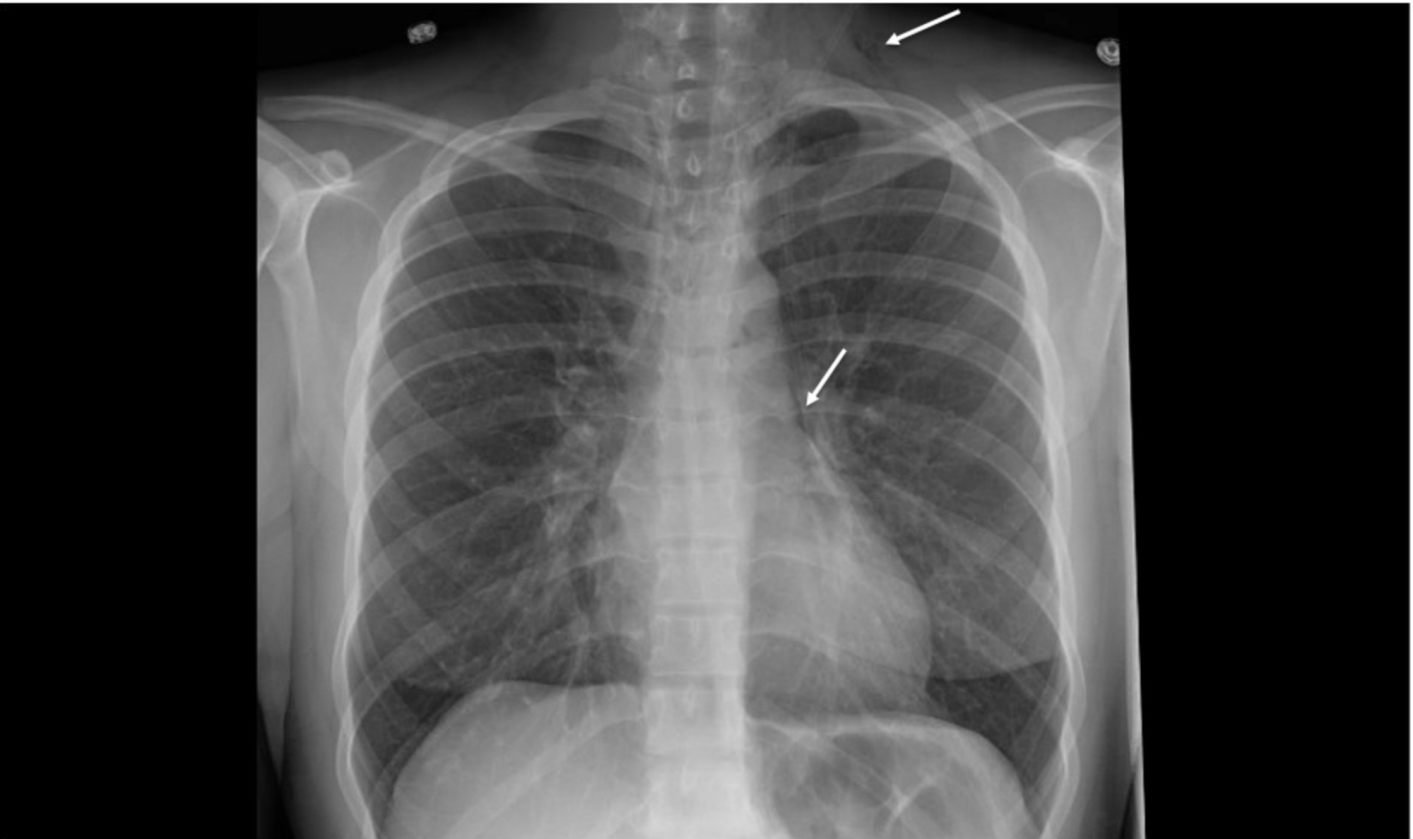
Plain chest radiograph demonstrating pneumomediastinum (linear lucency outlining left heart border - lower white arrow) and subcutaneous emphysema (lucency visualized in left lower neck - upper white arrow)
